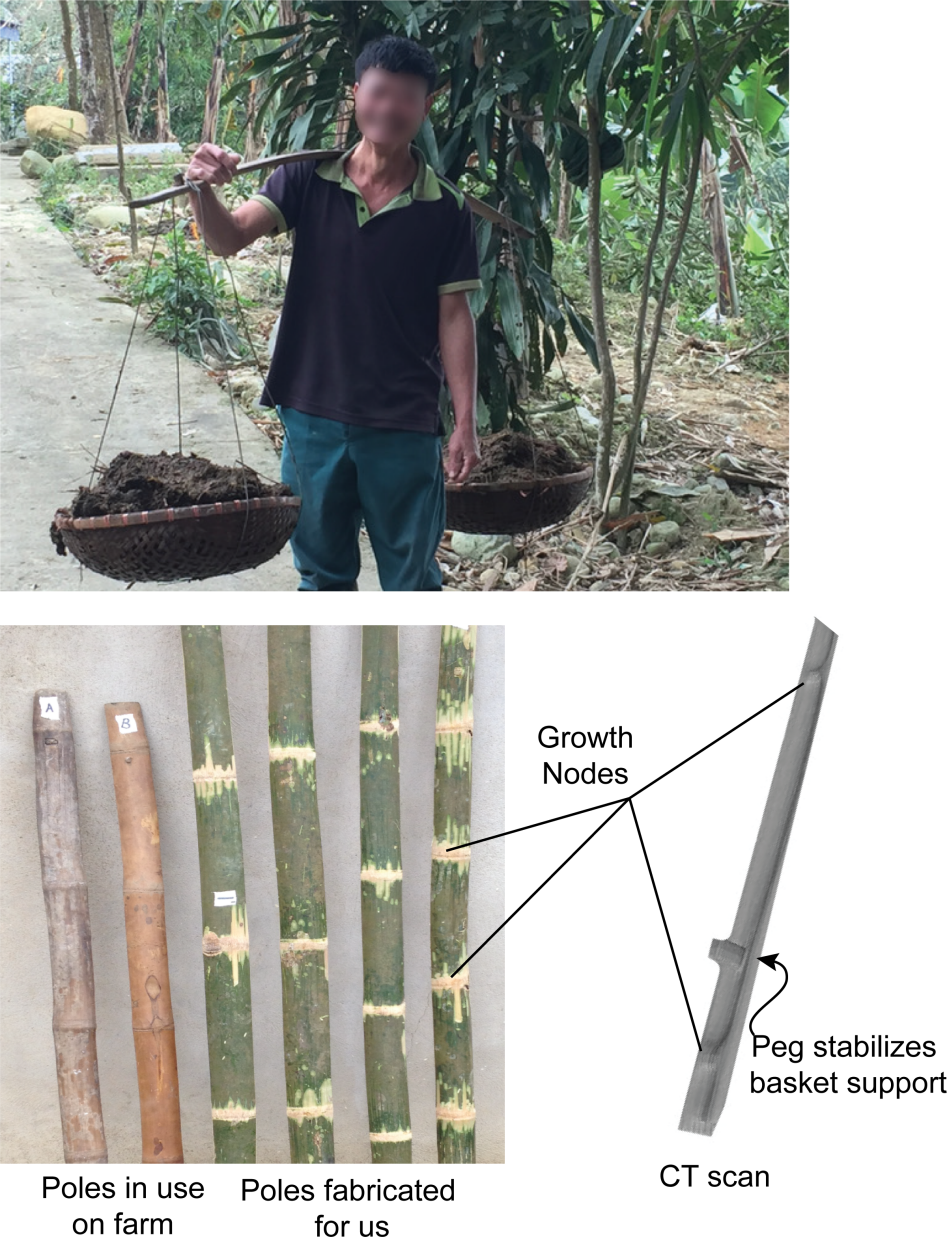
Pole carrying technique and example poles. Top: A farm worker carries a bamboo pole in northern Vietnam. The pole is supported at the shoulder with the hand (same side as the supporting shoulder) resting on top of the forward end to steady the system. Growth nodes are also indicated (Bottom Left: poles used in the study; Bottom Right: CT scan of pole C). These nodes are characterized by a thickening of the cross-section from a portion of a hollow tube to a portion of a solid cylinder.
